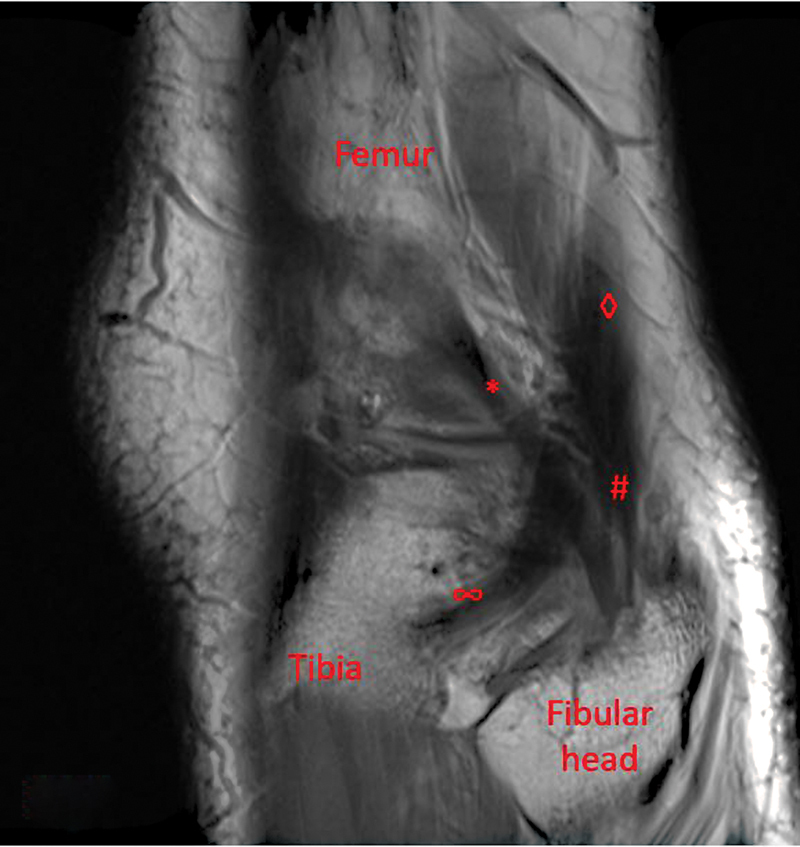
Magnetic resonance imaging showing lateral meniscus tear with flipped fragment (*), biceps femoris (◊), biceps femoris attachment into fibular head (#), and accessory attachment into tibia (∞).
